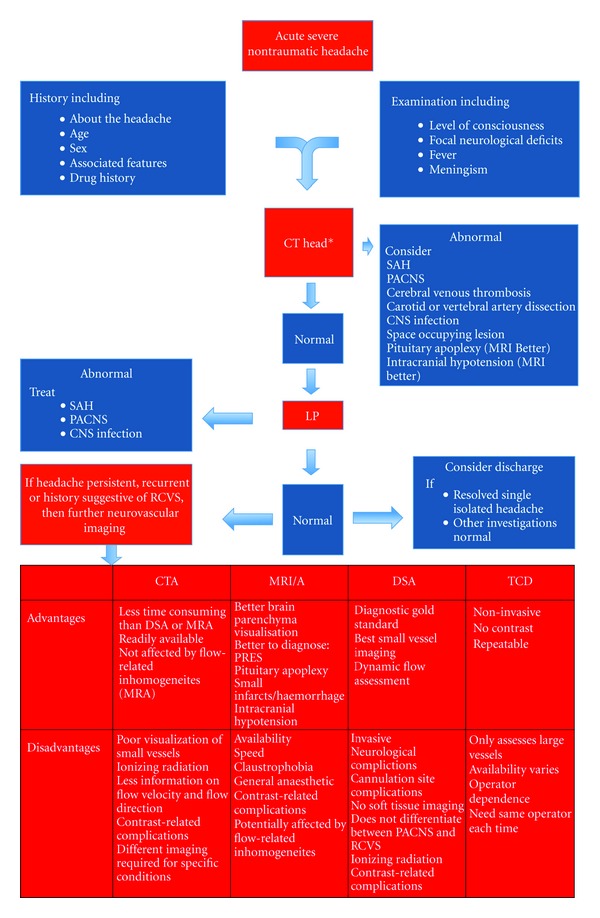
An approach to investigation of RCVS [10]. *CT angiography may be considered at this stage, specifically looking for cervical artery dissection, cerebral venous thrombosis or RCVS, depending on the history, clinical suspicion and contraindications to radiocontrast.
